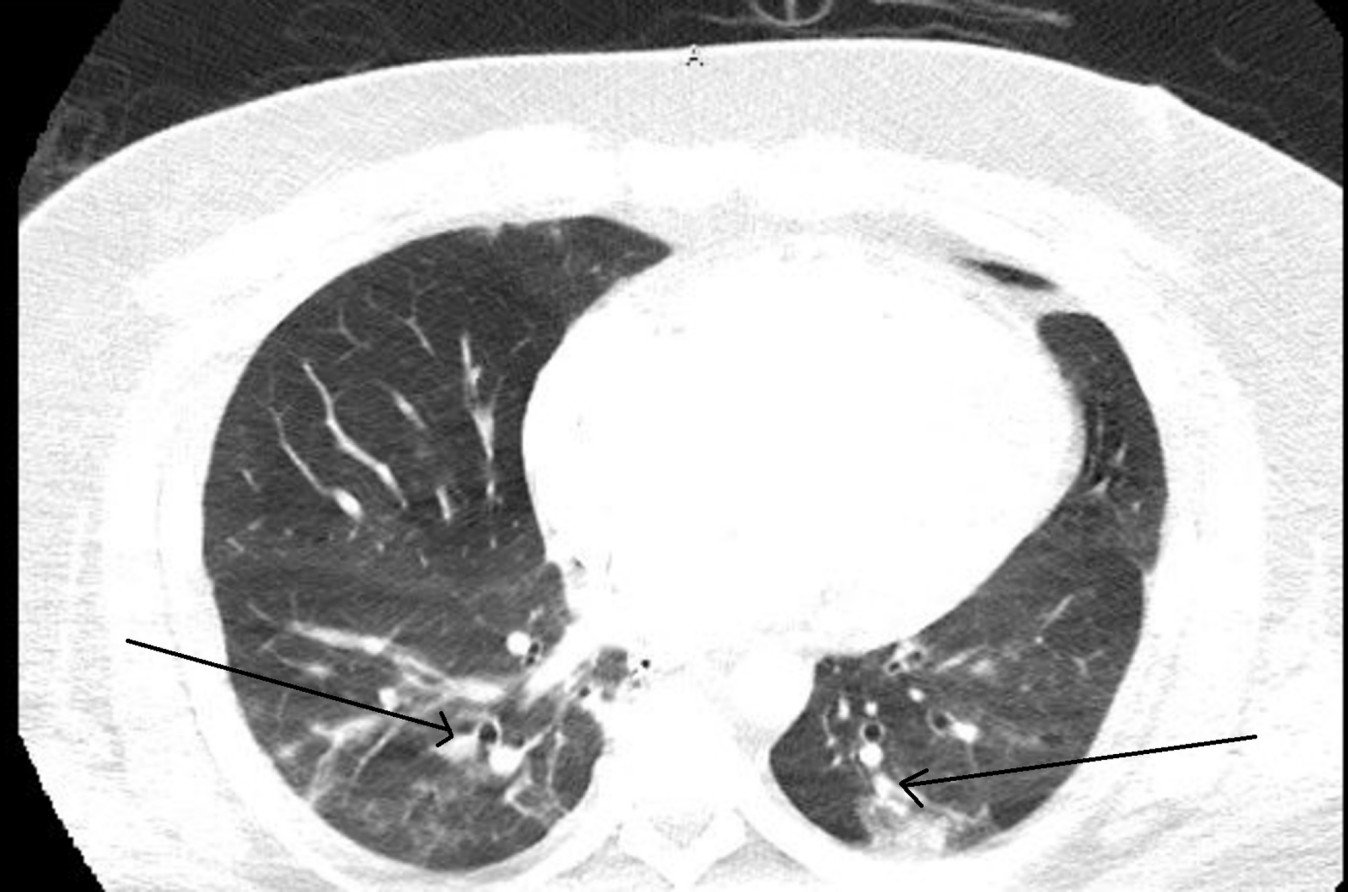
CT-Pulmonary angiogram of the chest, lung window Bilateral ground glass opacities are noted in the lungs bilaterally in addition to enlarged right atrium and ventricle
